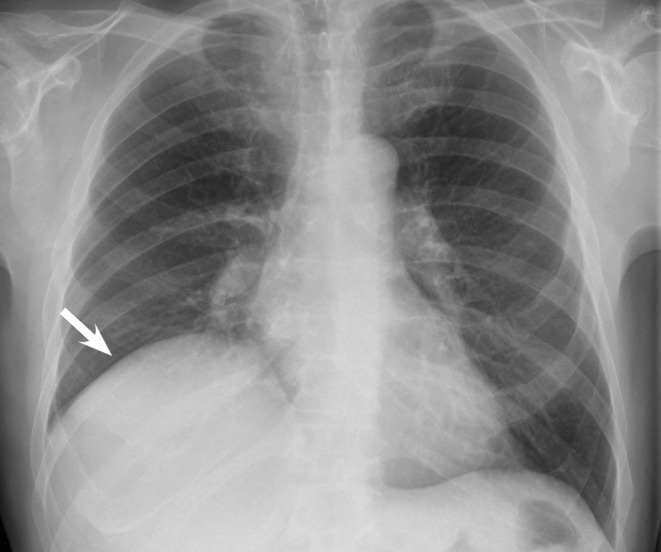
Upright chest x-ray demonstrating what seemed to be an elevated right hemidiaphragm (*arrow*).
